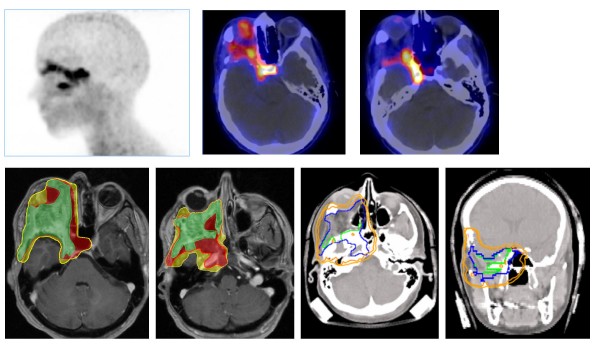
Large skull base meningioma with orbital invasion and close relation to the sella turcica region, [68Ga]-DOTATOC-PET (top left)/CT image fusion (top right). Physiological tracer uptake of the pituitary gland. CTV/GTV contours (below left): red = GTV-PET; green = GTV-MRI/CT; yellow = CTV, CTV enlargement by GTV-PET. Dose distribution with enclosing 90% PTV isoline.
